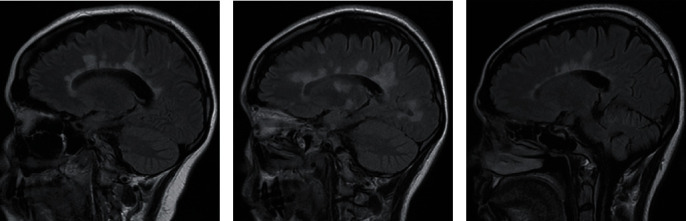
Sagittal FLAIR images from different MS patients in our center: (a) a 28-year-old female with RRMS, disease duration of 13 years; (b) a 29-year-old female with RRMS, disease duration of 1 year; (c) a 38-year-old female with RRMS, disease duration of 12 years, showing periventricular lesions with the characteristic “Dawson finger” appearance, indicating extension along the medullary veins.
